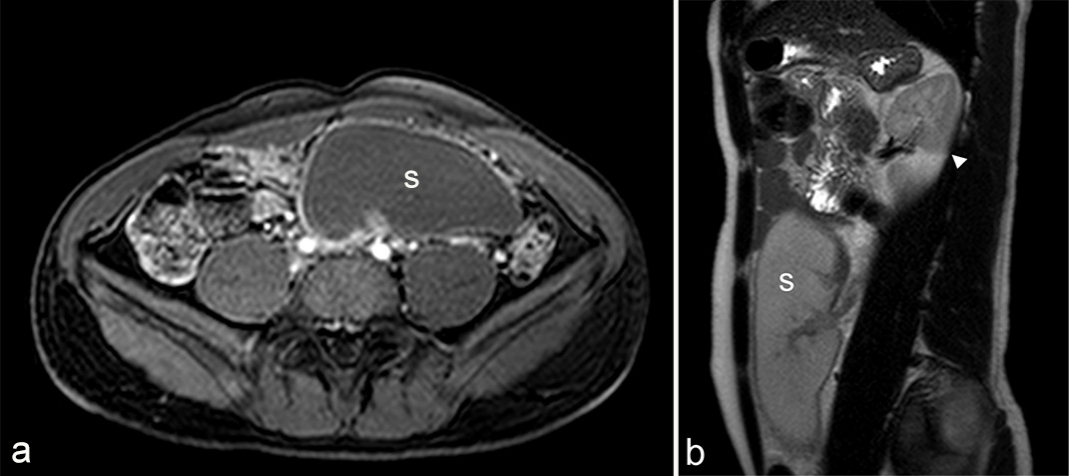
(a) Axial post-contrast *T*_1_ image showing the non-enhancing spleen and thin rim of enhancement of the splenic capsule located in the left lower quadrant (S). (b) Axial parasagittal *T*_2_ weighted MRI shows an ectopic spleen located under the left kidney (S). Note that the left kidney is slightly malrotated (white arrowhead).
